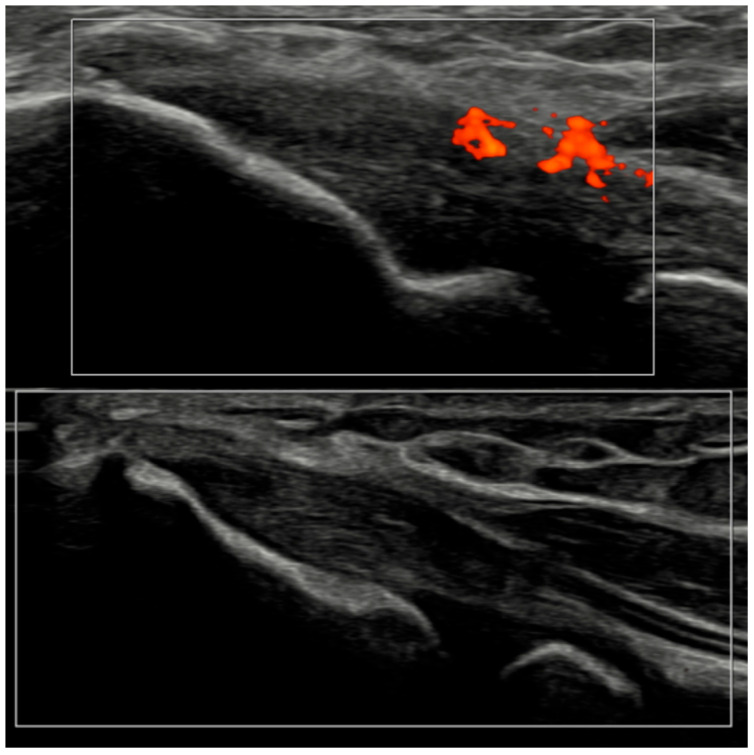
Ultrasound images of the elbow extensor enthesis at baseline (T0, top image) showing power Doppler signal (PD grade 2), and at 3 months (T3, bottom image), demonstrating resolution of power Doppler signal (PD grade 0).
